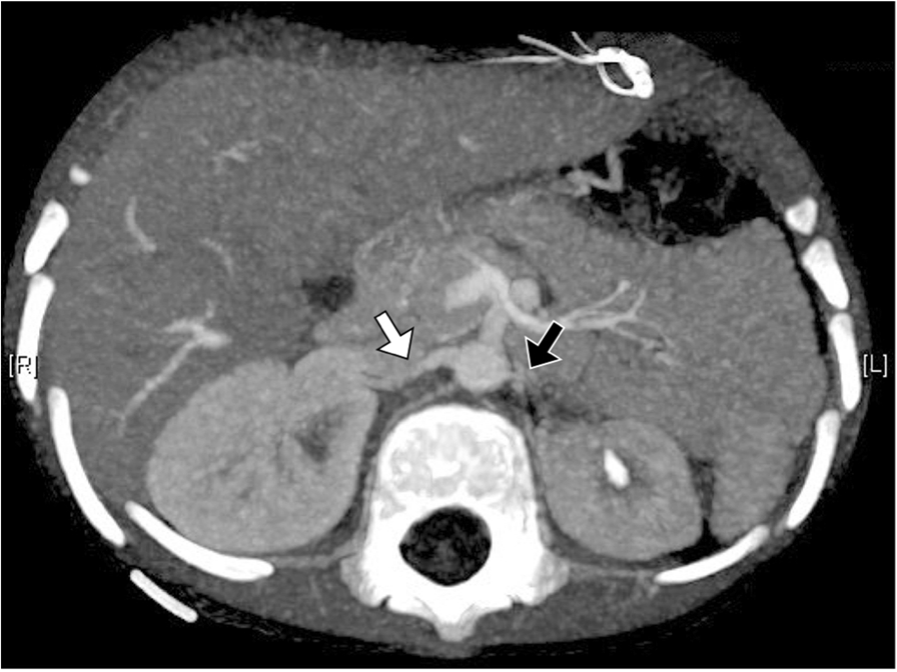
Computed tomographic angiography with maximum intensity projection. The axial view demonstrated the small caliber of the left renal artery (black arrow) with the compensatory change of the contralateral renal artery (white arrow)
